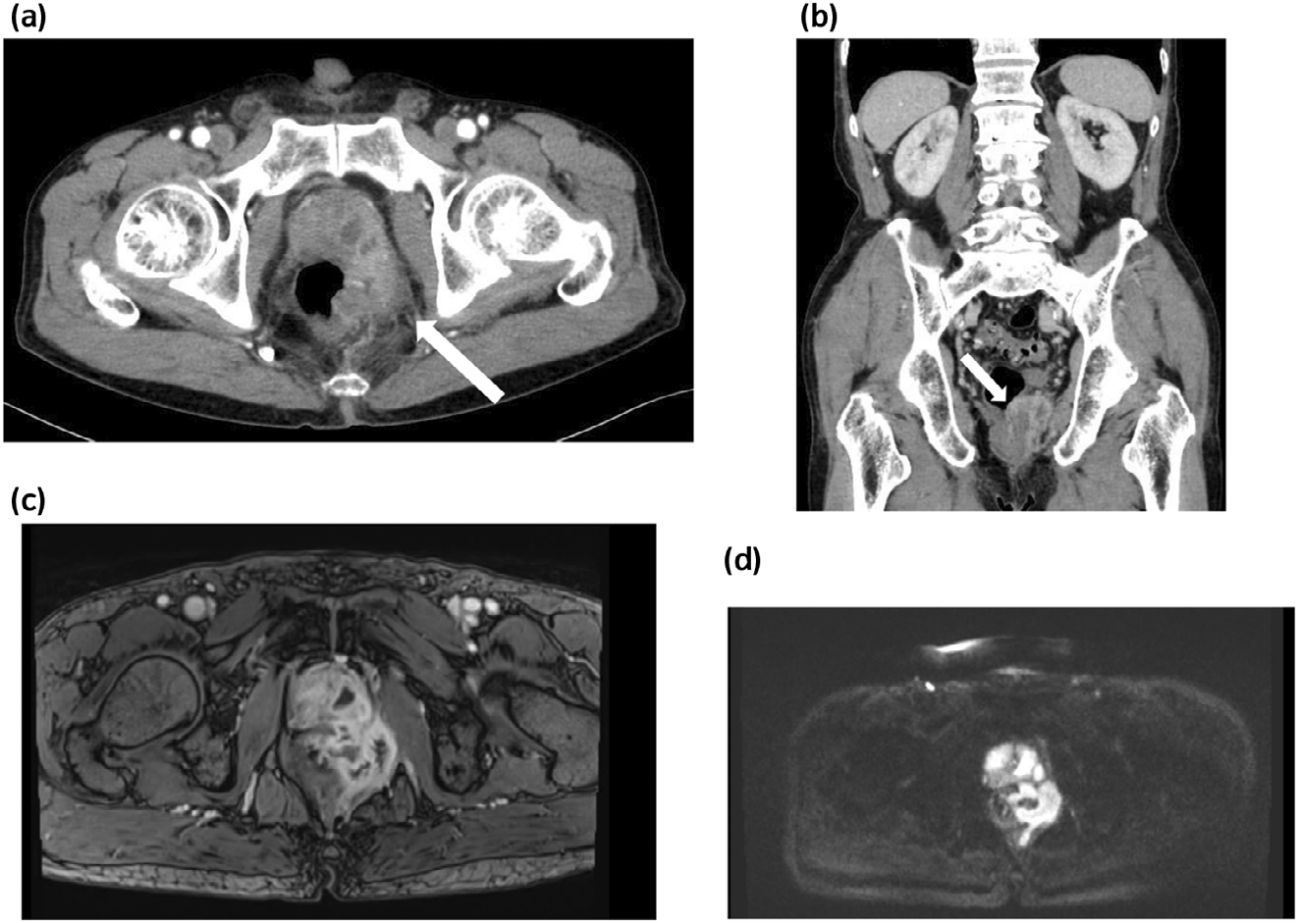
CT and MRI images of the pelvic mass. (a, b) The CT images showed mixed contrast enhancement and low‐density areas in the left prostate lobe, surrounding area and rectum (white arrows; a, axial view; b, sagittal view). (c, d) The MRI images showed contrast enhancement (c, T1‐weighted image) and reduced diffusion (d, diffusion‐weighted image) in the same area.
